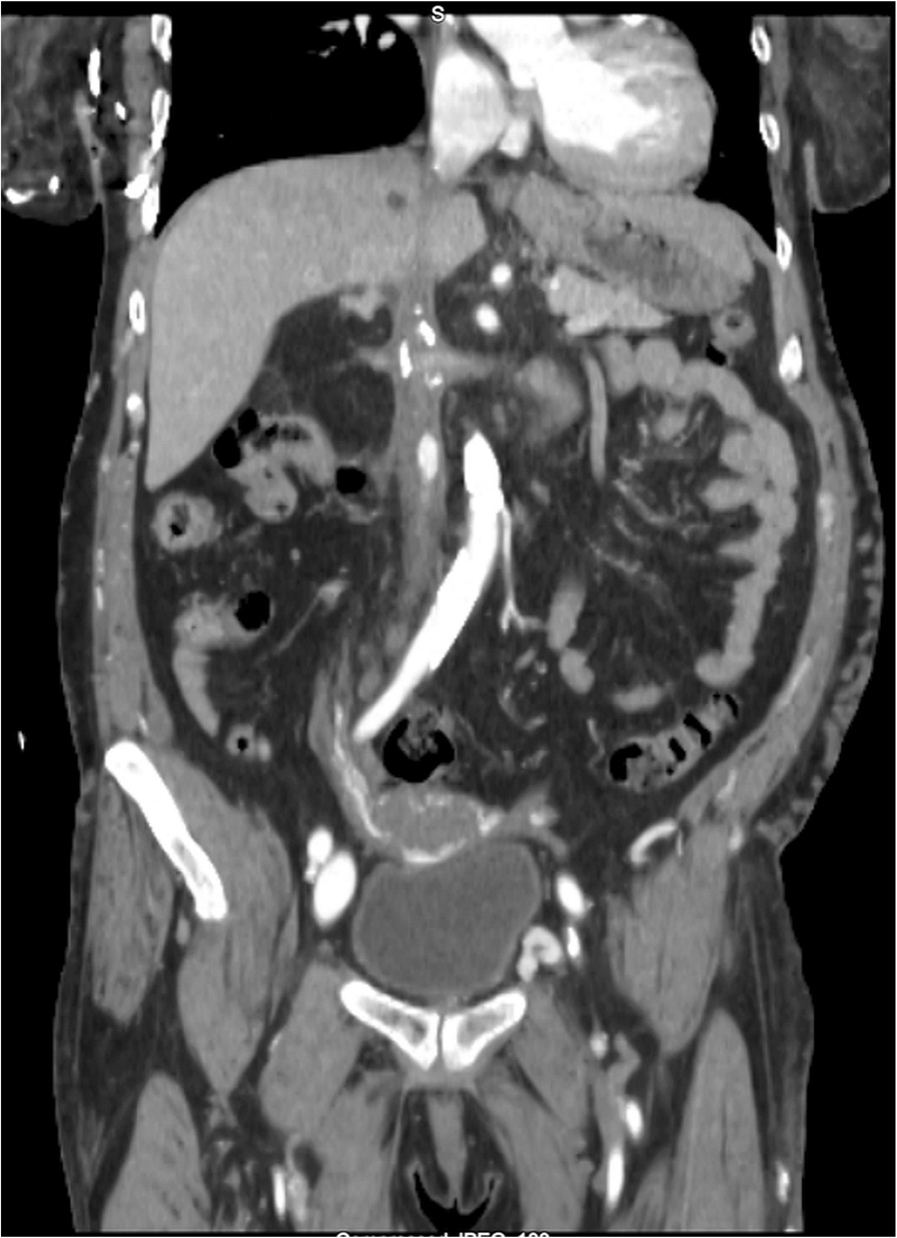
Initial CT scan showing calcified chronic occluded IVC and abdominal wall collaterals
